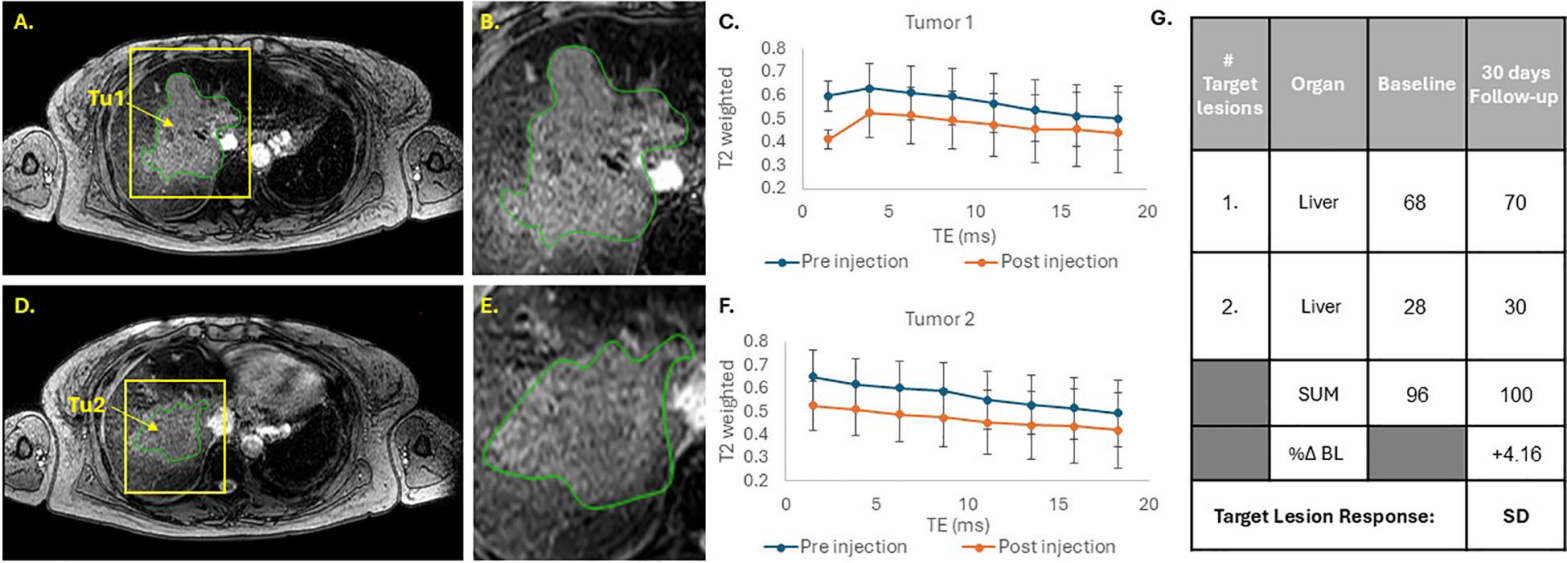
MRI analysis of SaNP accumulation in tumors of patient 2. T2*-weighted MR images (A, B, D, E). Yellow arrows indicate tumor locations. Tumors are marked in green. T2*-weighed signals of tumor Tu1 (C) and tumor Tu2 (F). Blue line – pre-injection (baseline); Orange line – post-injection (~4hrs.) and grey line – 30 days after treatment. Echo time (TE) was measured in milliseconds (ms). CT assessments and RECIST 1.1 evaluation (G).
